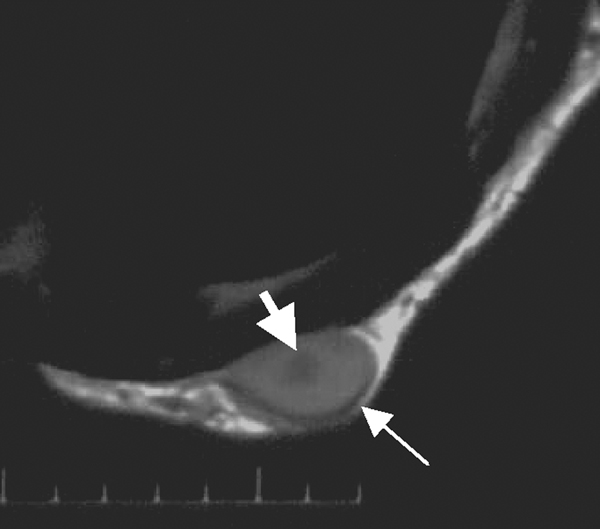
Axial T2-weighted magnetic resonance image shows a heterogeneous low signal mass with a low signal central region (upper arrow) and between the edge of which and the surrounding fat tissue there is a district rim with low signal intensity (lower arrow).
